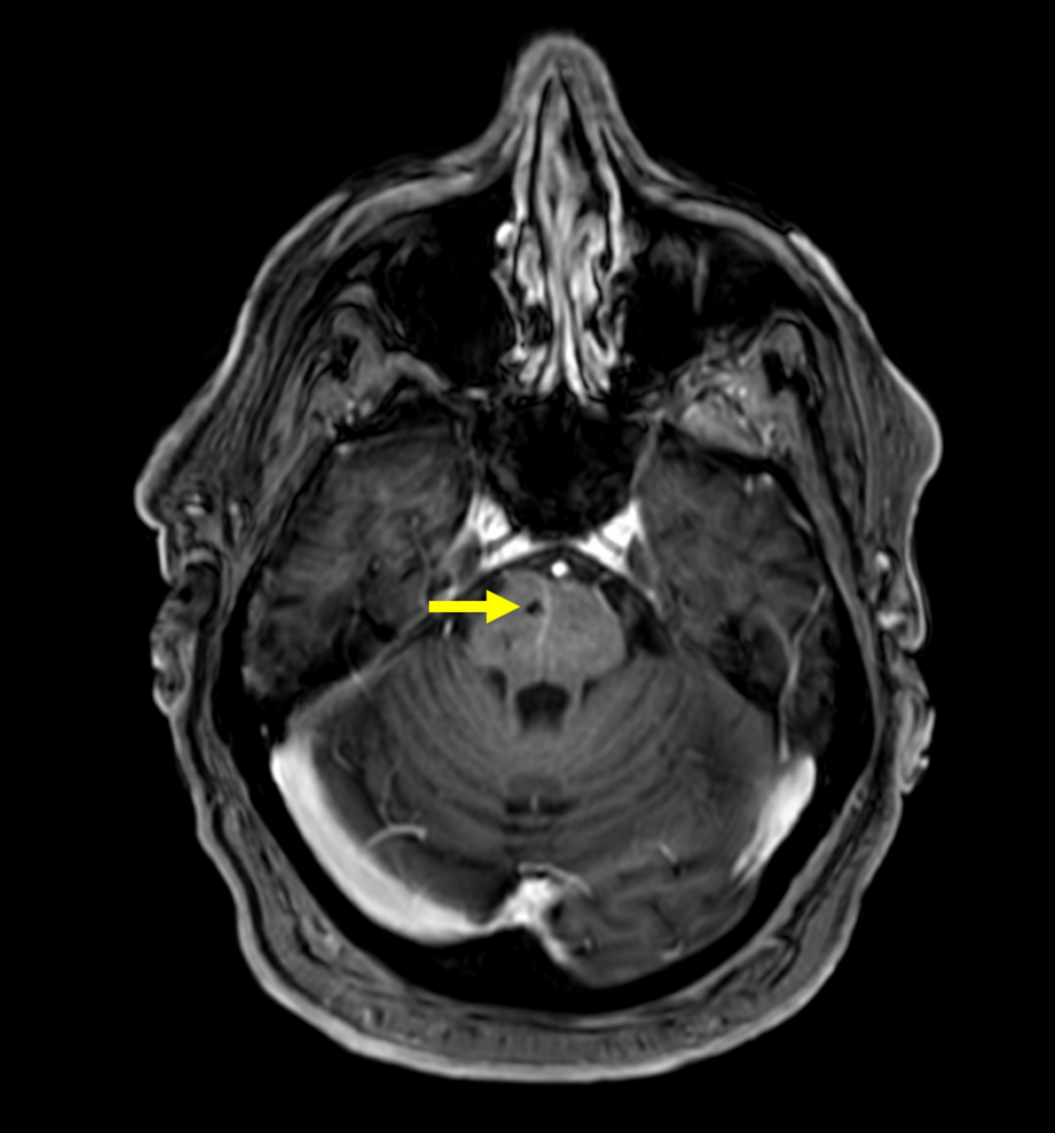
MRI of the brain showing small arteriovenous malformation in right pons (yellow arrowhead)
